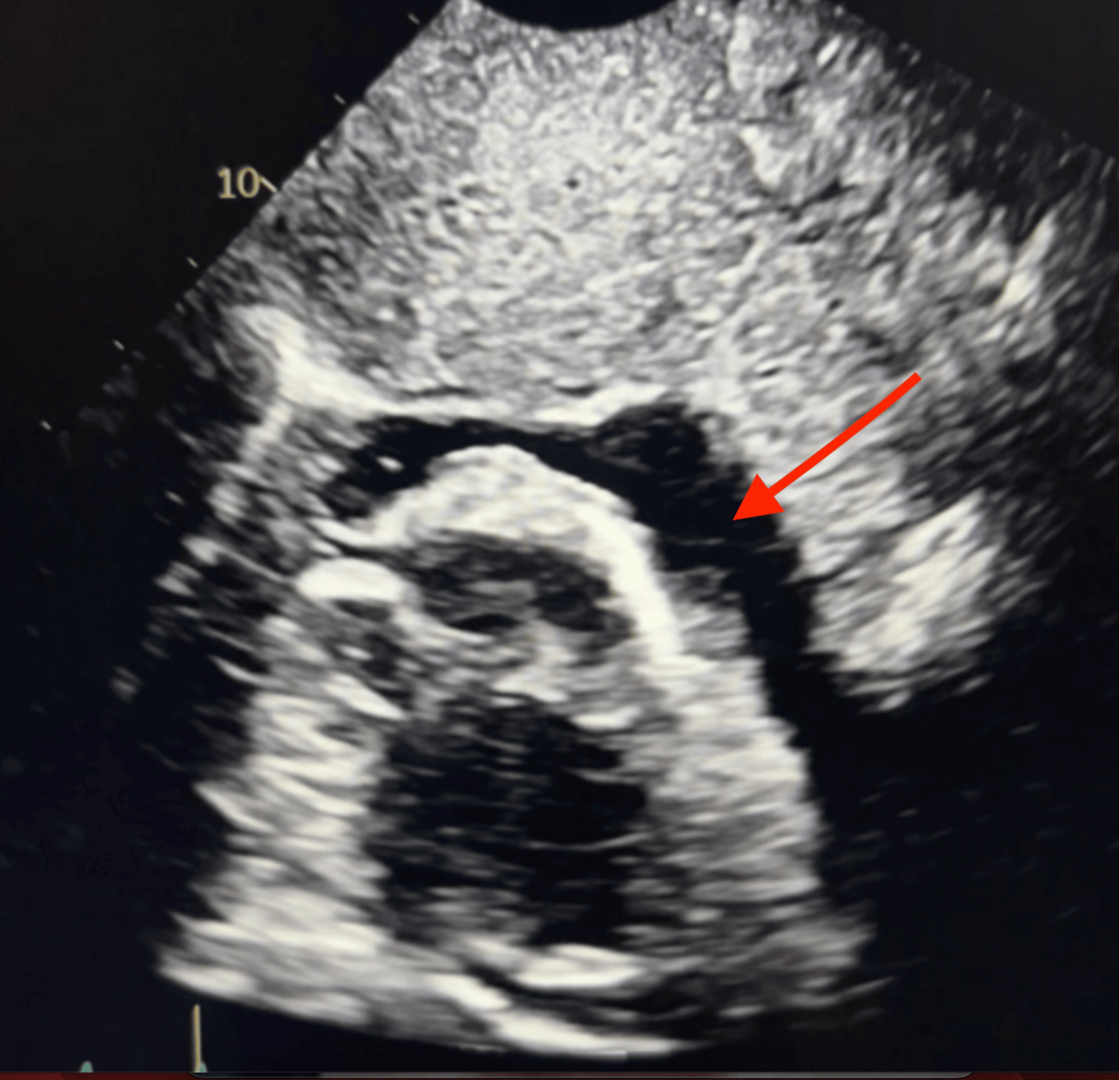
Two-dimensional (2D) echocardiography obtained in the emergency department (ED), demonstrating large pericardial effusion
